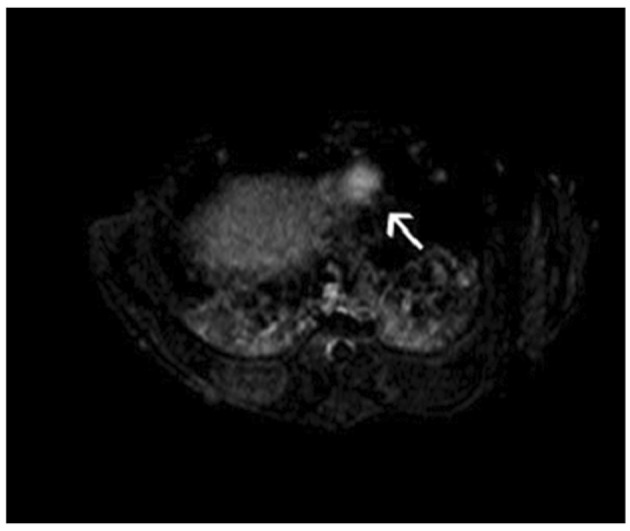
MRI showed a high-intensity signal for the VX2 tumor (white arrow) on diffusion images, allowing clear visualization of the boundary. No necrosis with low-intensity signals was detected for the VX2 tumor prior to treatment.
